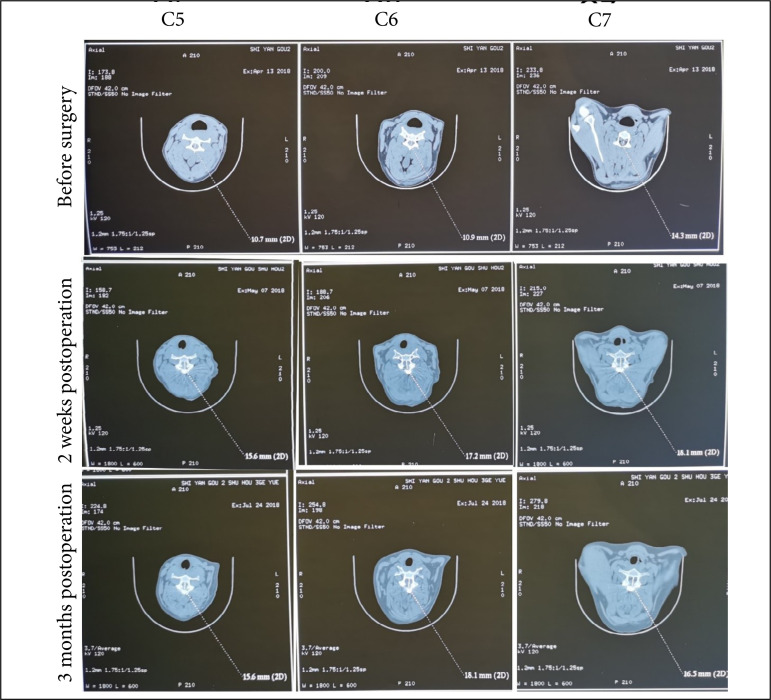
The CT measurement on the anteroposterior diameter of the spinalcanal (C5–C7) before surgery and at 2 weeks and 3 months after operation.
